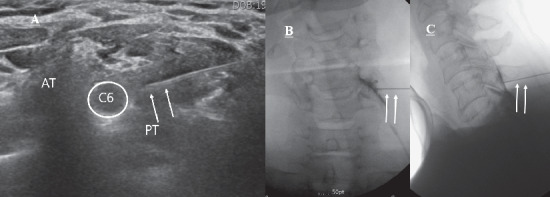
(A) Ultrasonography-guided selective nerve root block (target nerve root: C6). The needle (arrow) is placed on the dorsal surface of the C6 nerve root. AT, anterior tubercle; PT, posterior tubercle. (B) C6 transforaminal epidural injection. The A-P view of the contrast media which spread into the intraforaminal lesion. (C) C5-6 interlaminar epidural injection. Lateral view of the contrast media which spreads into the epidural space. The arrow indicates a needle.
